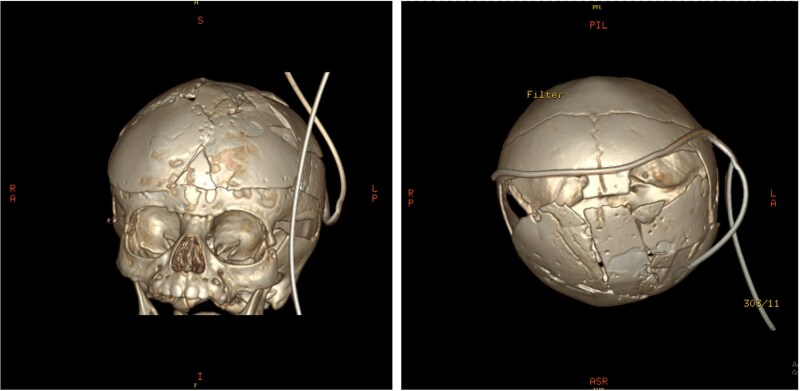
Post-operative 3D reconstruction CT showing skull shape correction and cosmetic results.
